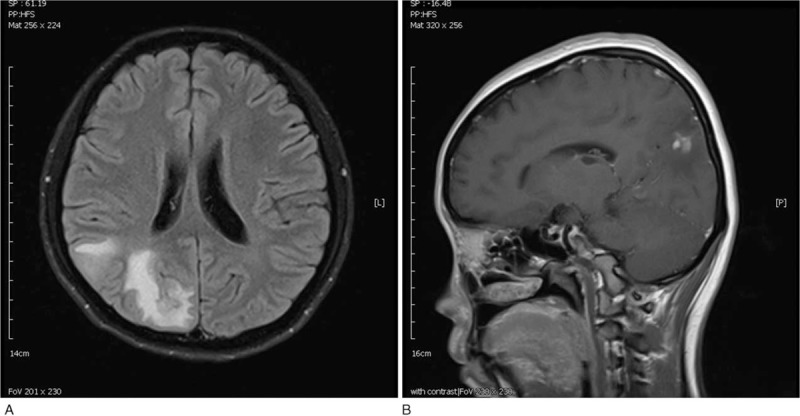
Brain magnetic resonance imaging showed disseminated septic emboli and microabscess.
